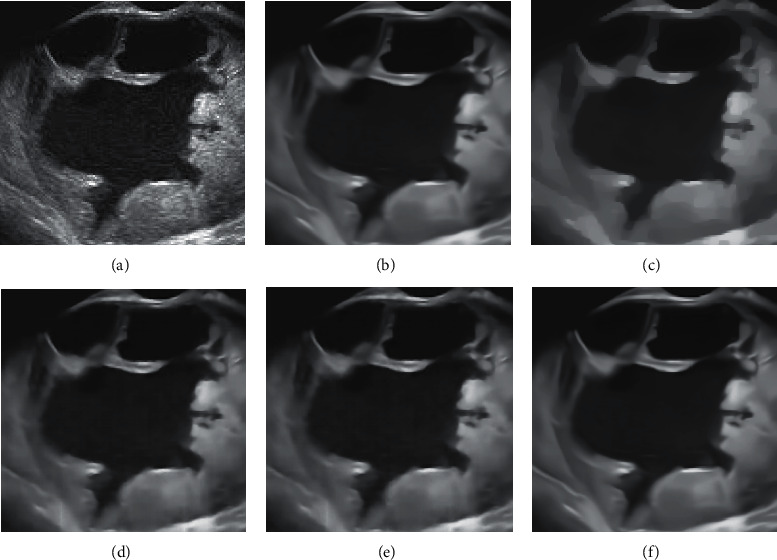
Results of real ovarian cancer ultrasound images. (a) The original image. (b) The results of this model. (c) VA results. (d) SRAD results. (e) RED-Net results. (f) DnCNN results.
